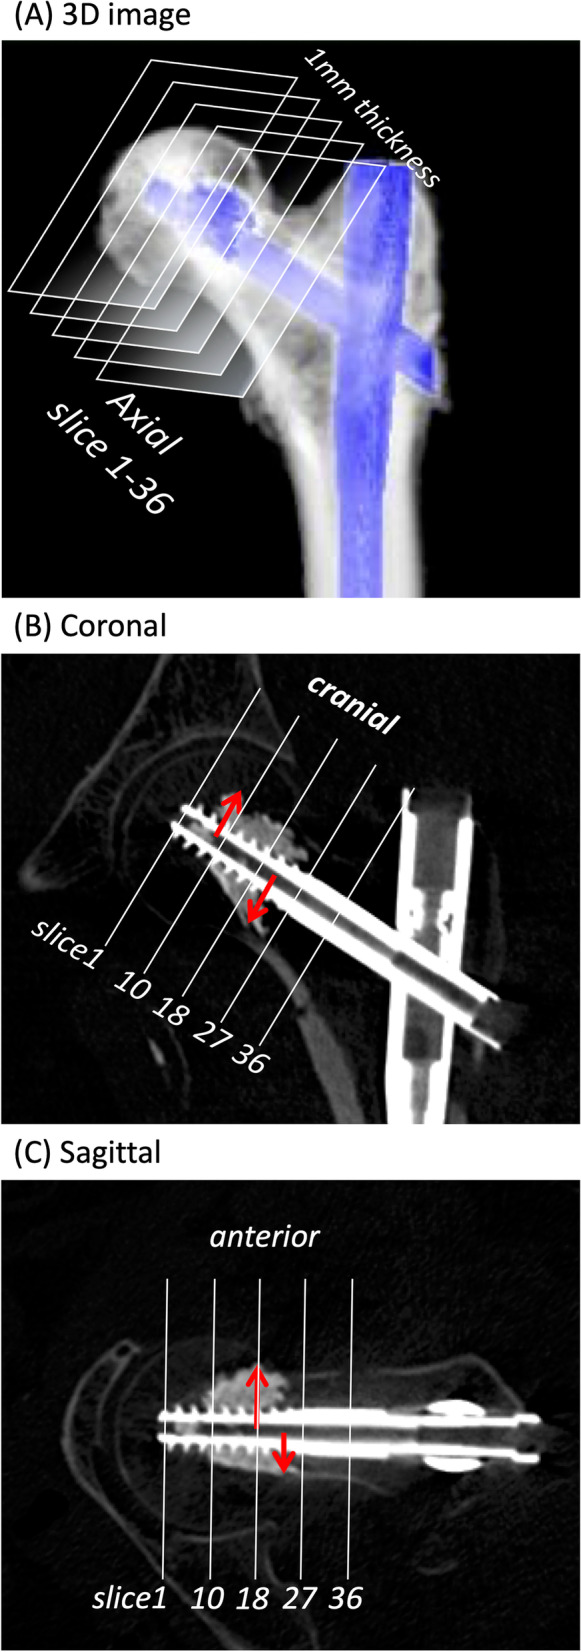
Radiographic parameters using coronal and sagittal CT. A 3D image: The head element was cut into round slices in the axial plane: 36 successive 1-mm slices starting at the tip of the head element (slice 1). B Coronal plane: where the head element and the inserted nail were placed in the same plane. The maximum penetration depth (MPD) (cranial and caudal) was measured as the distance from the center of the head element to the end of cement distribution (red arrow). C Sagittal plane: perpendicular to the coronal plane and parallel to the head element. The MPD (anterior and posterior) was measured (red arrow). The picture above is a lag screw case
